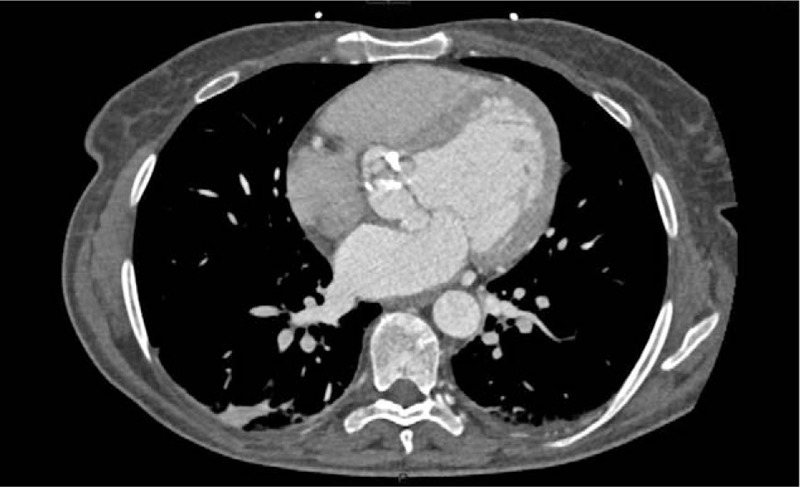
Extended aortic valve calcification at CT chest scan.
